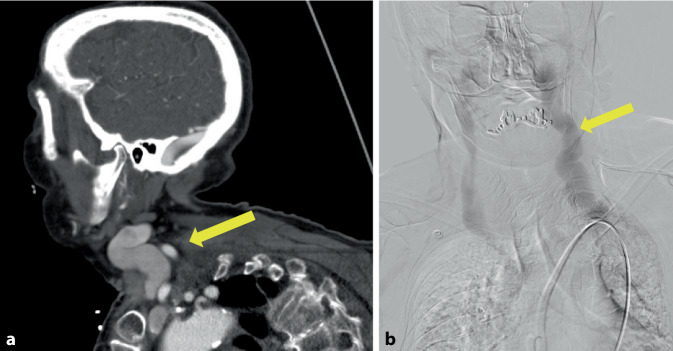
Fall 4: Aneurysma der V. jugularis interna links. a CT-Angiographie in der venösen Phase und sagittaler Schichtung mit Darstellung der aneurysmatischen V. jugularis interna links (*gelber Pfeil*), b Venöse digitale Subtraktionsangiographie der aneurysmatischen linken V. jugularis interna (*gelber Pfeil*)
